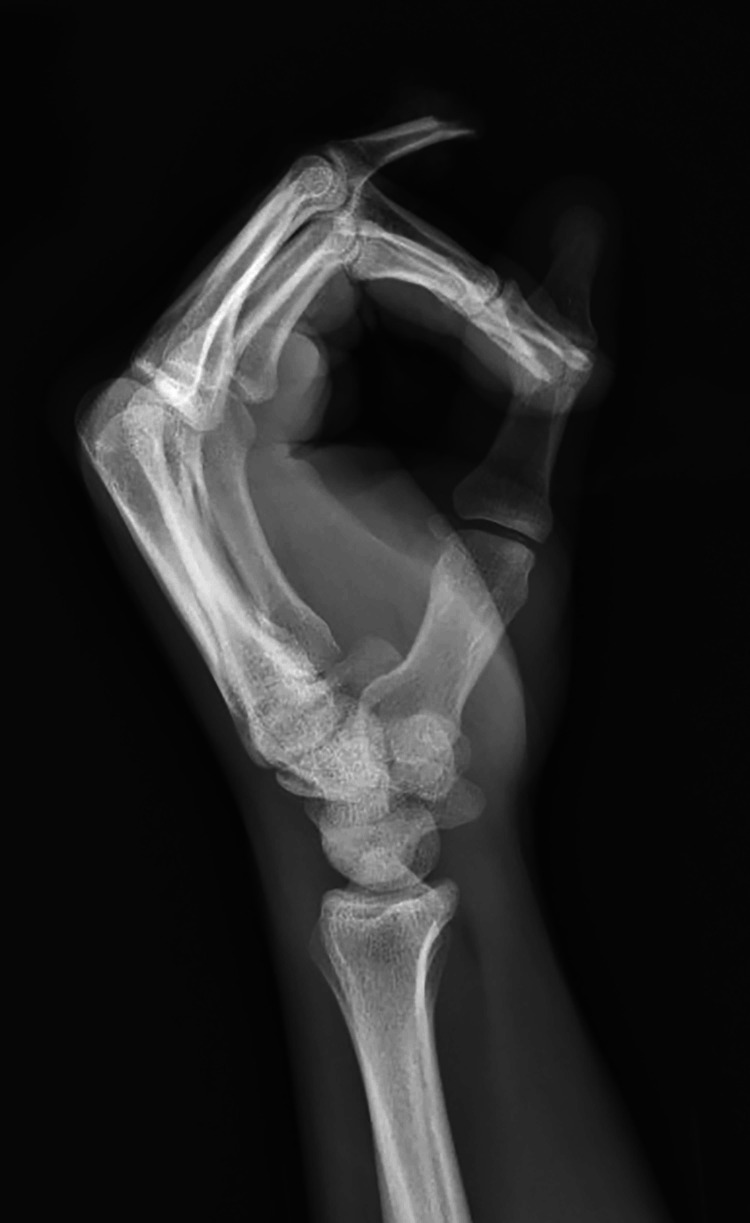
Lateral plain radiograph of the left hand demonstrating amputation through the ring finger middle phalanx.
